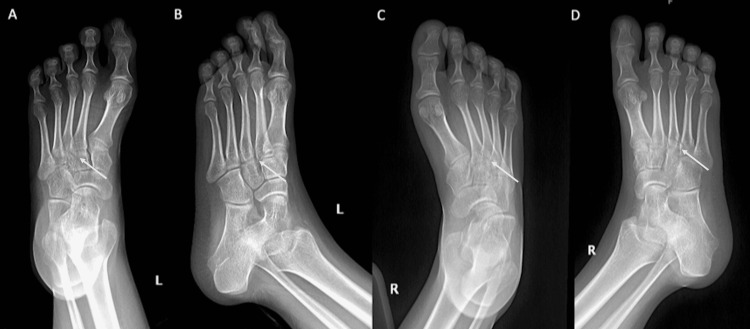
Anteroposterior and oblique x-ray views of both feet at the time of injury (A) Left foot AP x-ray. (B) Left foot oblique x-ray. (C) Right foot AP x-ray. (D) Right foot oblique x-ray. AP: Anteroposterior.
